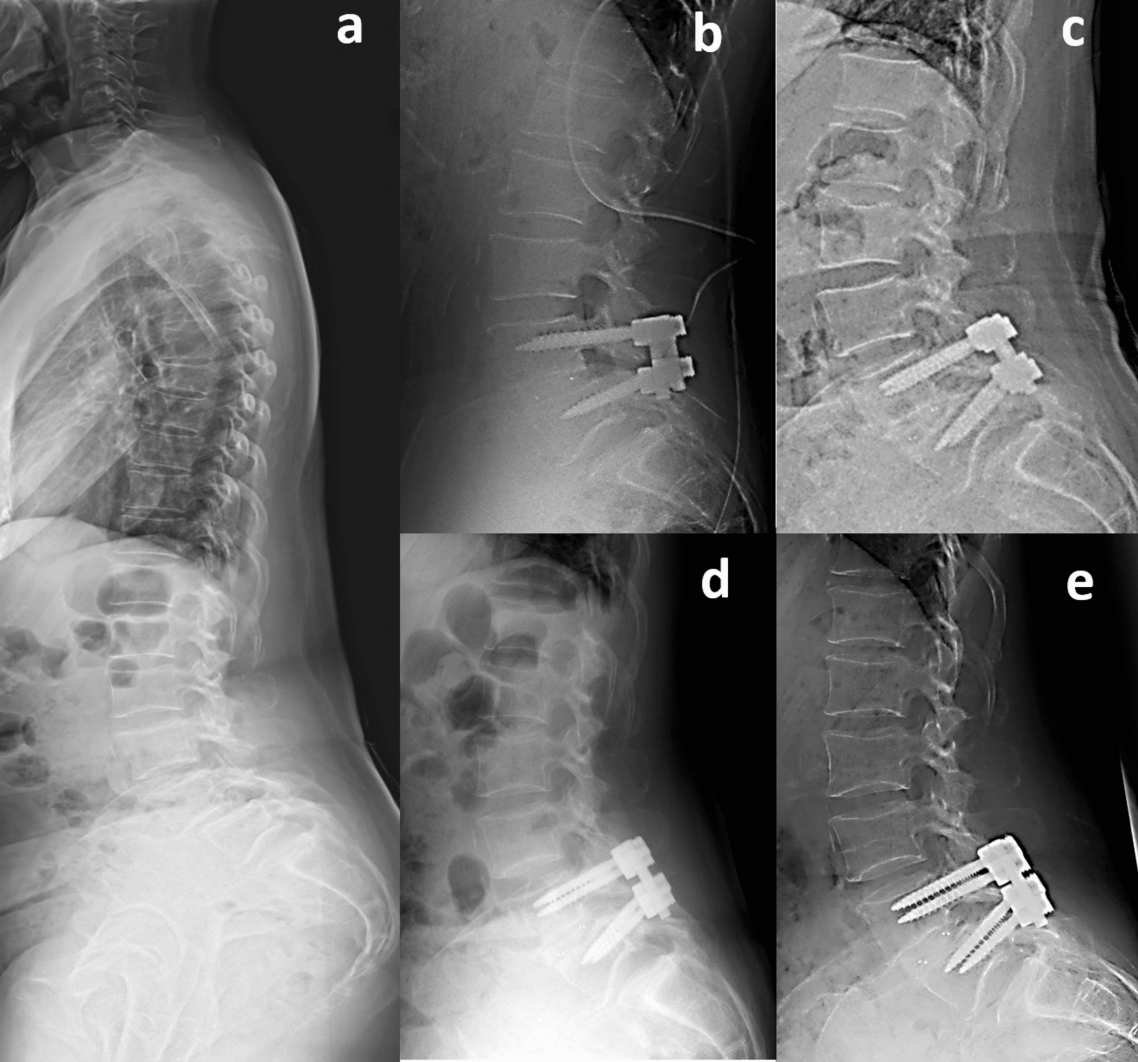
Pre and postoperative radiographs of Case 1 a. Preoperative sagittal whole-spine radiograph: lumbar lordosis (LL) 43˚, pelvic incidence (PI) 94˚, pelvic tilt (PT) 48˚, sagittal vertical axis (SVA) 108mm, L4 segmental lordosis 23 ˚ and L4-SVA 68mm. b. Immediate postoperative lateral lumbar radiograph: L4 segmental lordosis 33˚. c. Lateral lumbar radiograph at fracture onset: LL 40˚ and L4-SVA 62mm. d. Lateral lumbar radiograph after early ambulation. e. Lateral lumbar radiograph at final follow-up.
